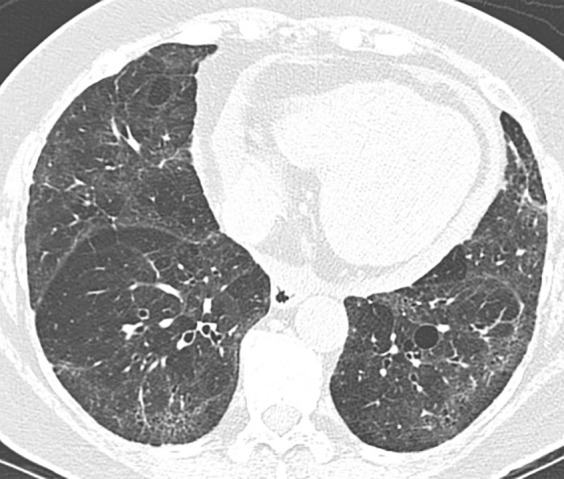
Nonspecific interstitial pneumonia. (a) Axial CT scan demonstrates fibrotic nonspecific interstitial pneumonia early in the disease course with lower lung fibrosis and ground-glass opacity with some subpleural sparing. (b) Axial CT scan 10 years later demonstrates substantial progression of the pulmonary fibrosis, which now demonstrates increased reticular abnormality, less ground-glass abnormality, and more traction bronchiectasis. Understanding the evolution of the disease by reviewing the prior imaging is critical in these cases.
